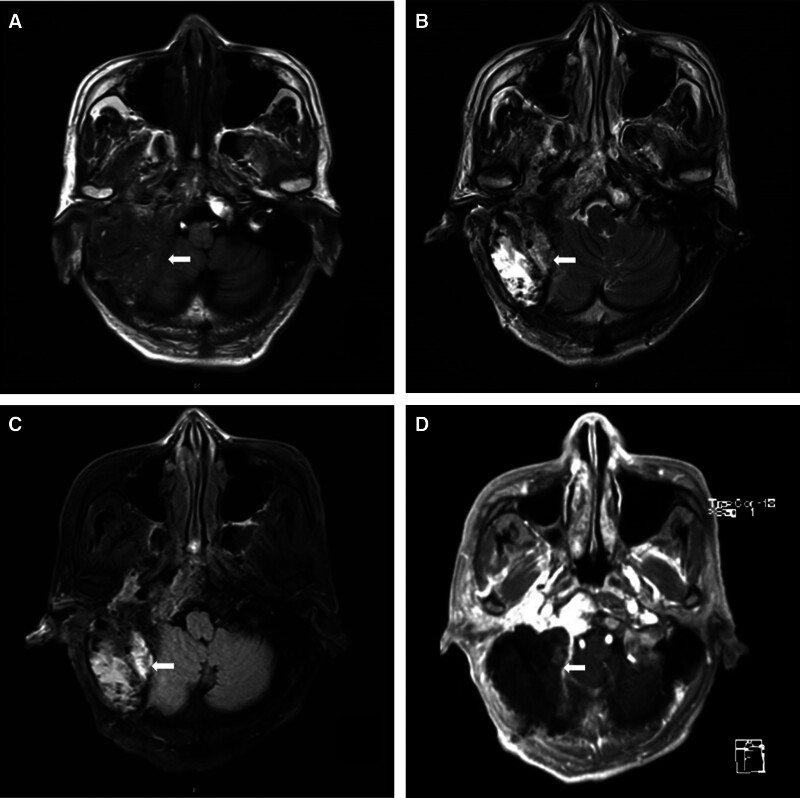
Postoperative magnetic resonance imaging revealed irregular heterogeneous T1 (A) and T2 (B) signal intensity in the operated area, heterogeneous signals intensity in the FLAIR sequence (C), and no obvious abnormalities was seen in the enhancement scan (D).
